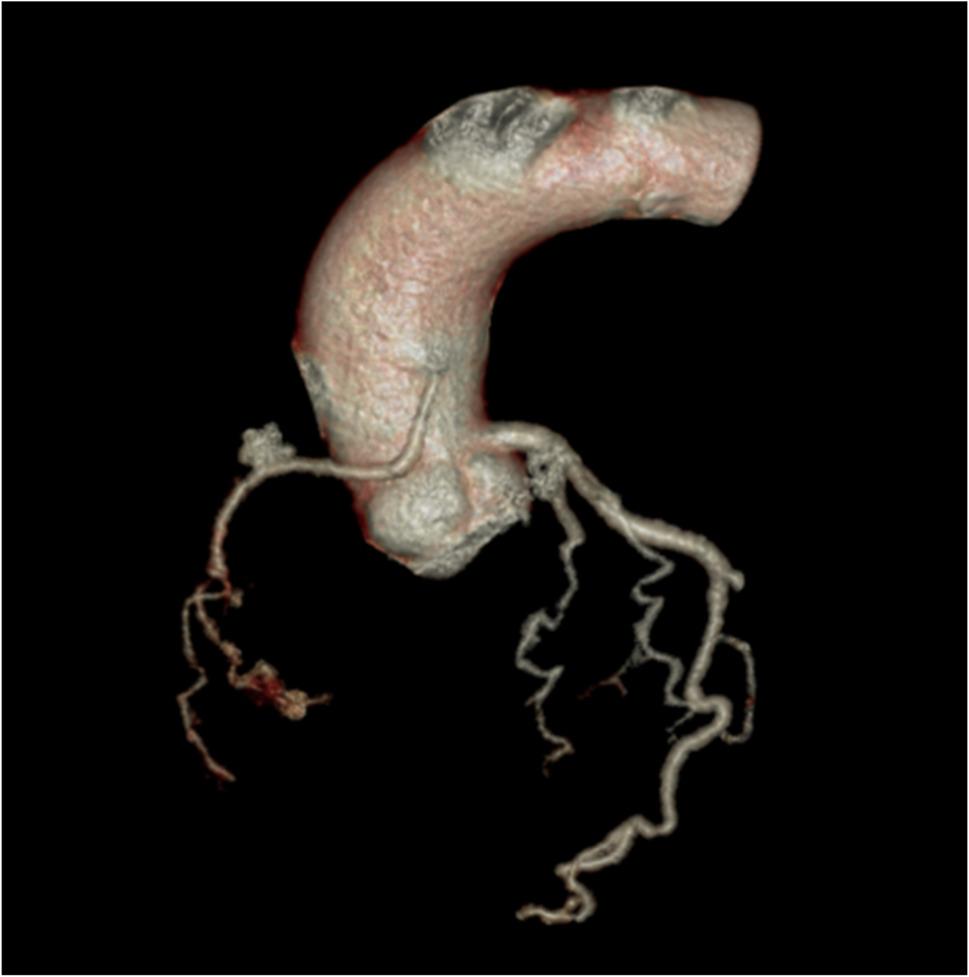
Computed tomography coronary angiography confirming anomalous origin and course. Three-dimensional volume-rendered computed tomography coronary angiography showing anomalous origin of the right coronary artery from the anterior and left lateral wall of the ascending aorta with a short interarterial course between the ascending aorta and the main pulmonary artery
